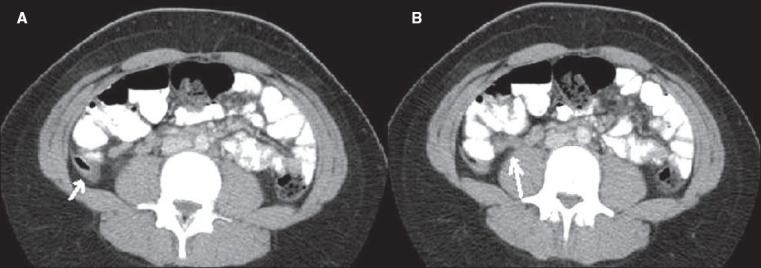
Mesenteric adenitis. Contrast-enhanced CT scan shows enlarged mesenteric lymph nodes (long arrow in A) in the right lower quadrant mesentery. The appendix is normal (short arrow in B)
